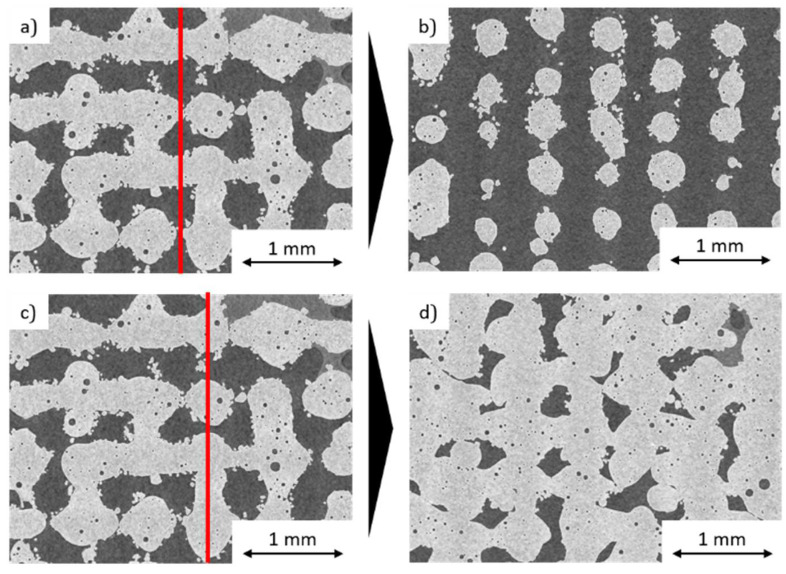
CT-Image: (a) top view, (b) sectional side view, (c) top view, (d) sectional side view of a lattice-structure (P_L_ = 385 W, v_s_ = 500 mm/s, g_w_ = 0.3 mm, l_s_ = 250 µm).
